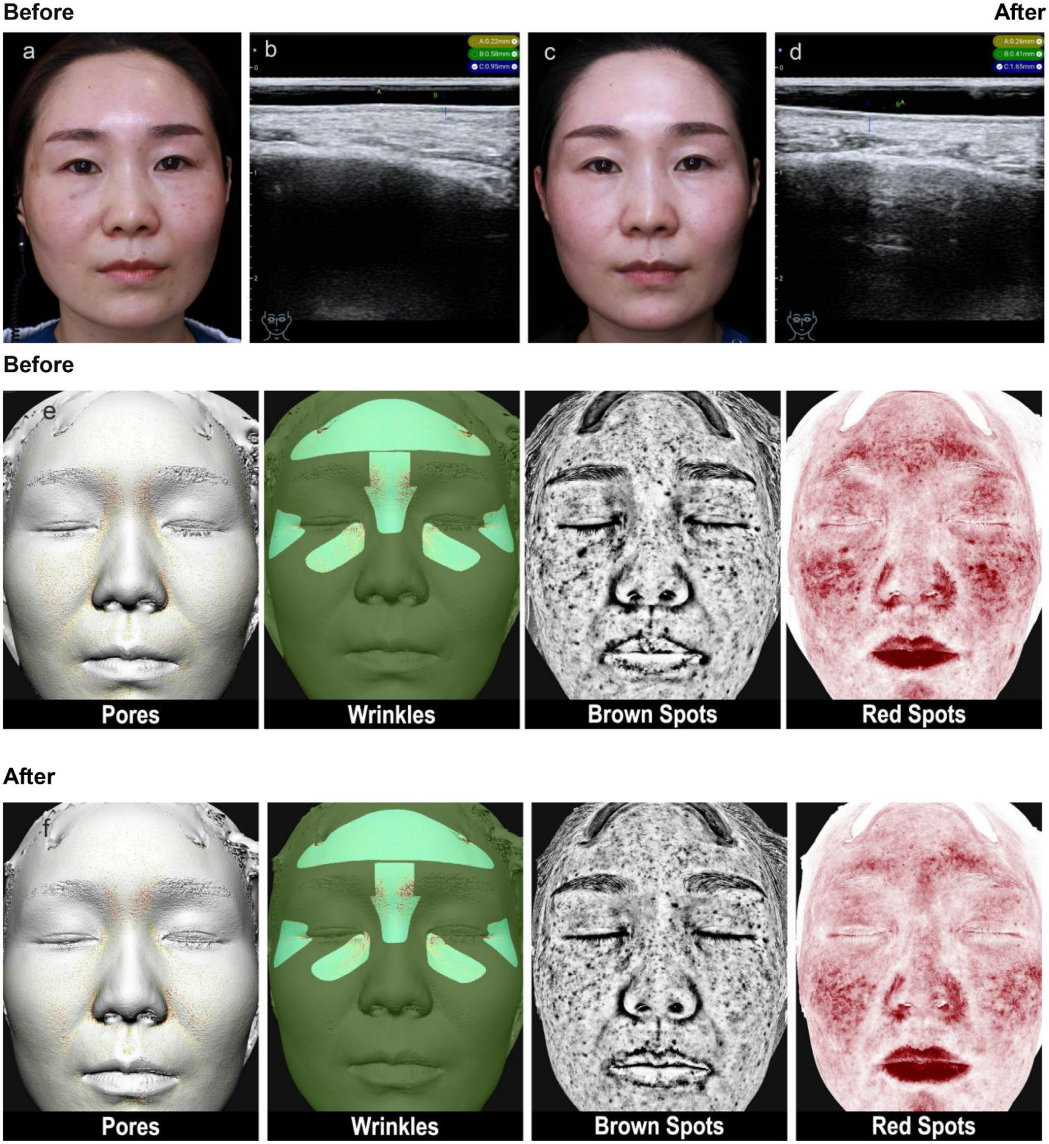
Typical case 3. “38‐year‐old female, sensitive and red skin, thin skin, dynamic wrinkles around the eyes.” (a) “Clinical image before PRP treatment.” (b) “High‐frequency ultrasound (HFUS) measurement of epidermis, SLEB, and dermal thickness in the infraorbital area.” (c) “Clinical image four weeks after the third PRP treatment.” (d) “HFUS measurement of skin thickness in the infraorbital area four weeks after the third PRP treatment.” (e, f) “Skin analyzer images of Pores, Wrinkles, Brown Spots (superficial pigmentation, deep pigmentation, spot area), Red Spots before and four weeks after the third PRP treatment.”
